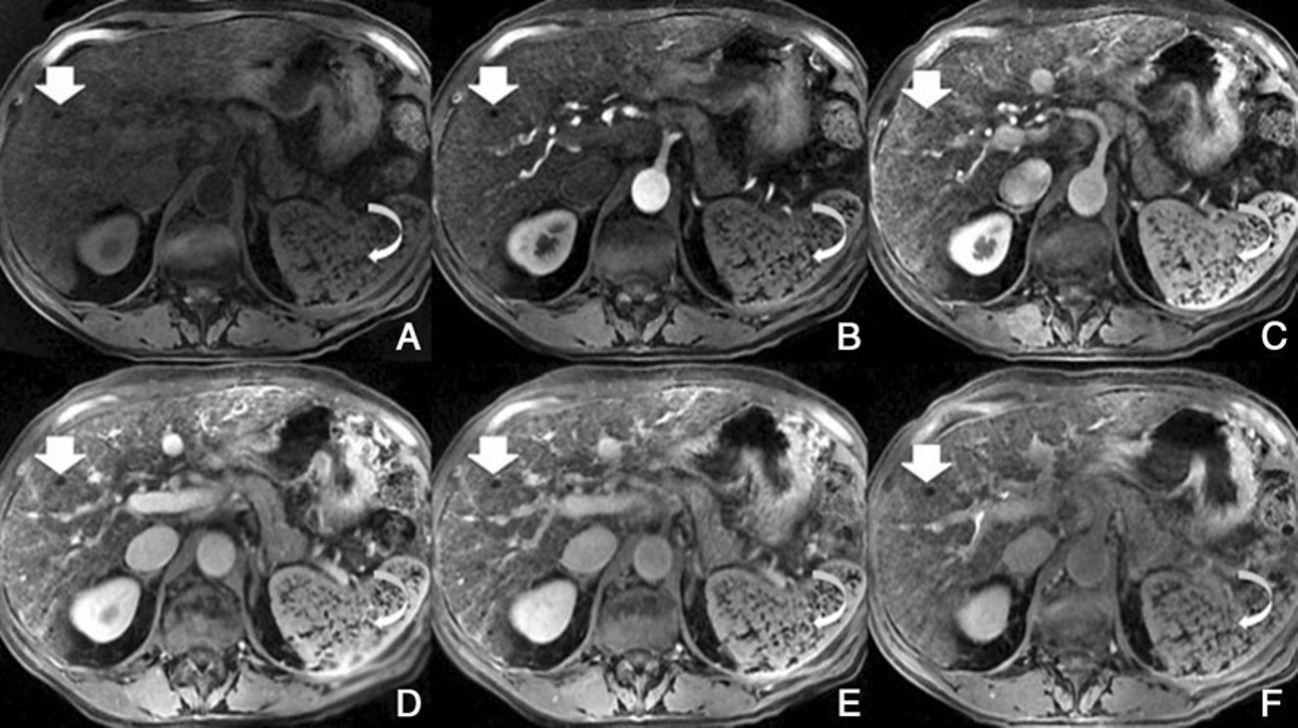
A benign cirrhosis-associated nodule in a 38-year-old woman with chronic liver disease. a Pre-contrast MRI, b, c arterial phase, d PV phase, e delayed phase, f hepatocellular phase. The image shows a liver with a lobulated contour and a nodular parenchyma (cirrhosis). The *solid arrows* point to a small rounded focal lesion, markedly hypointense in all sequences (more conspicuous with the increase of the TE), showing no enhancement with the contrast agent. The *curved arrow* points to multiple Gamna-gandy bodies (hemosiderin deposition)*Dynamic imaging with extracellular contrast agent*: After the injection of the contrast, most benign cirrhosis associated-nodules show similar enhancement to the adjacent liver in the arterial and venous phases [9, 10]. Some benign cirrhosis associated-nodules enhance in the arterial phase and typically fade to isointensity in the venous phases.*Imaging with Gd-EOB-DTPA*: Uptake and excretion of gadobenate dimeglumine by these nodules is usually preserved. Consequently, they are usually isointense in the hepatocellular phase (HCP) [11].*Differential diagnosis*: The diagnosis of most benign cirrhosis-associated nodules is straightforward with MRI, using extracellular agents. However, some benign cirrhosis associated-nodules may show enhancement in the arterial phase. These hypervascular benign nodules usually fade to isointensity in the venous phases. While most cirrhosis-associated nodules with arterial phase hyperenhancement and venous phase isoenhancement are benign, small hepatocellular carcinomas (HCCs) can have a similar appearance. Nodules with arterial phase hyperenhancement and venous phase isoenhancement can therefore cause diagnostic confusion and lead to inappropriate management. Use of Gd-EOB-DTPA can be helpful in the differential diagnosis of these lesions. Homogeneous isointensity in the HCP relative to liver suggests benignancy: routine follow-up is sufficient. By comparison, hypointensity in the HCP suggests malignancy: such lesions require biopsy, empirical treatment or close follow-up depending on lesion size and clinical considerations.*Potential pitfalls*: Siderotic and steatotic nodules may appear hypointense in the HCP, potentially mimicking early HCC. This is due to the iron and fat content being already of low signal intensity in the pre-contrast fat saturated sequences. Some benign nodules may appear hyperintense in the HCP [14] (Fig. 3). The mechanism is still unknown, but overexpression of organic anionic transporter peptides (OATP) or down-regulation of MDRs could possibly play a role. Such lesions may be mistaken for focal nodular hyperplasia (FNH)-like lesions (these are also benign, so the distinction is irrelevant) or atypical HCCs with HCP hyperintensity. The differentiation between benign and malignant HCP-hyperintense nodules is discussed in the following two sections. Small benign cirrhosis-associated nodules with arterial-phase hyperenhancement and HCP hypointensity may be indistinguishable from small HCCs.
